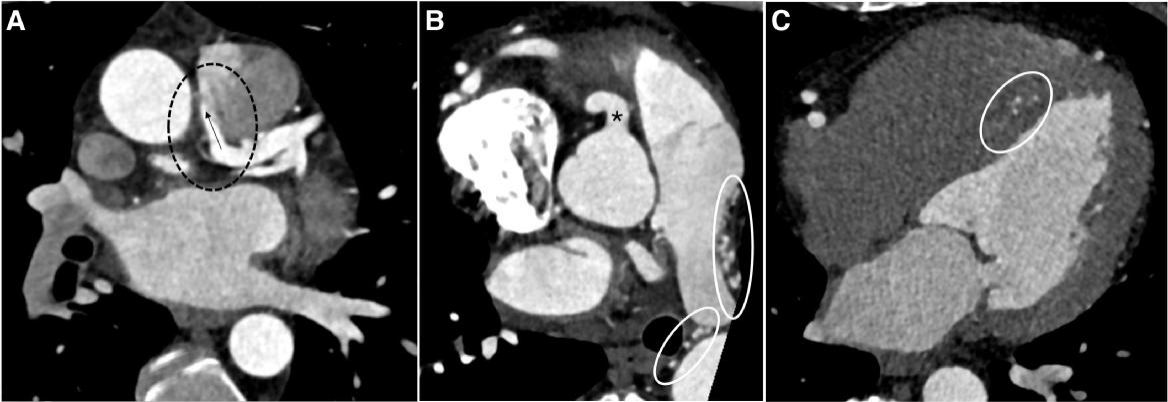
Coronary computed tomography angiography (CCTA). (*A*) Anomalous origin of the left coronary artery from the posteromedial side of the pulmonary trunk (black dashed circle) with contrast spillover into the pulmonary artery (black arrow). (*B*) Correct position of the origin of the right coronary artery (asterisk). Note multiple mediastinal collaterals (white circles) fed by bronchial arteries. (*C*) Prominent septal collaterals (white circle) fed by the right coronary artery.
